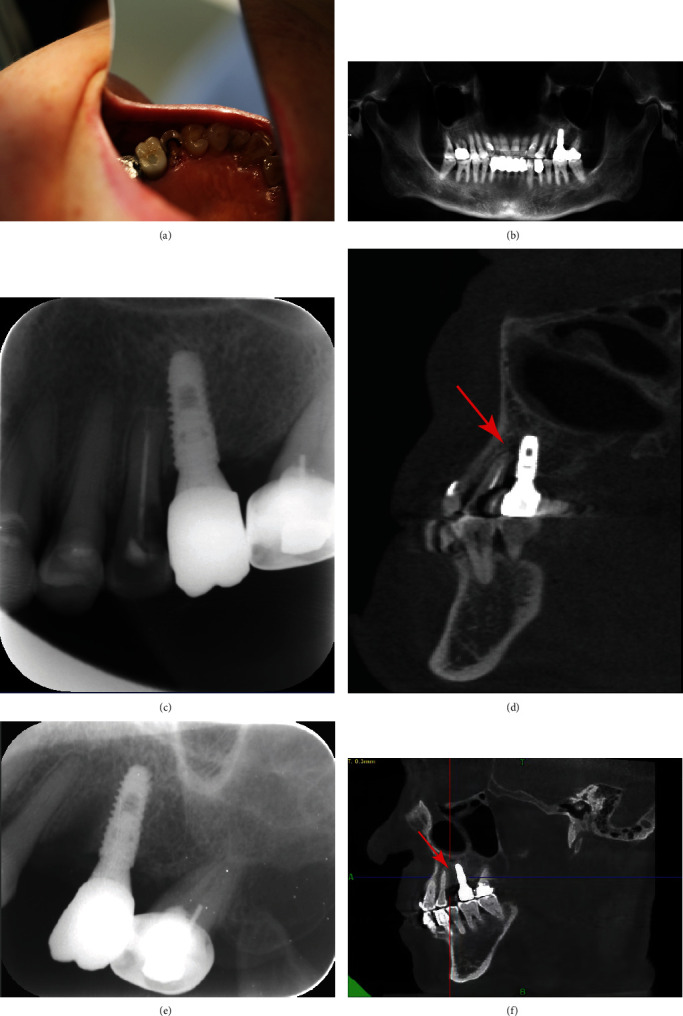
Case 1: (a) Preliminary photograph showed a defect of the palatal crown to the subgingival at tooth #25. (b) The original Cone-beam CT. (c) The apical radiograph revealed the insufficient distance between tooth #25 and the implant. (d) The coronal radiograph clearly showed that the apical lesion of tooth #25 destroyed the peri-implant bone (red arrow). (e) Three months after extraction. (f) Intraoral radiography one year after extraction (red arrow).
